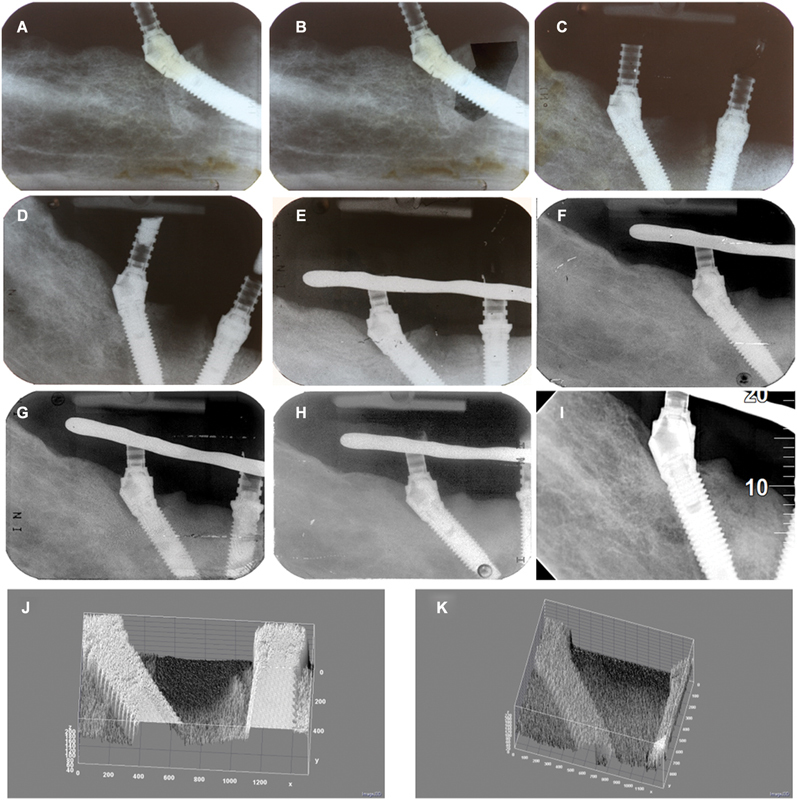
Radiographic control of the case between baseline and 18-year post-surgery between implants #42 and #45. (
